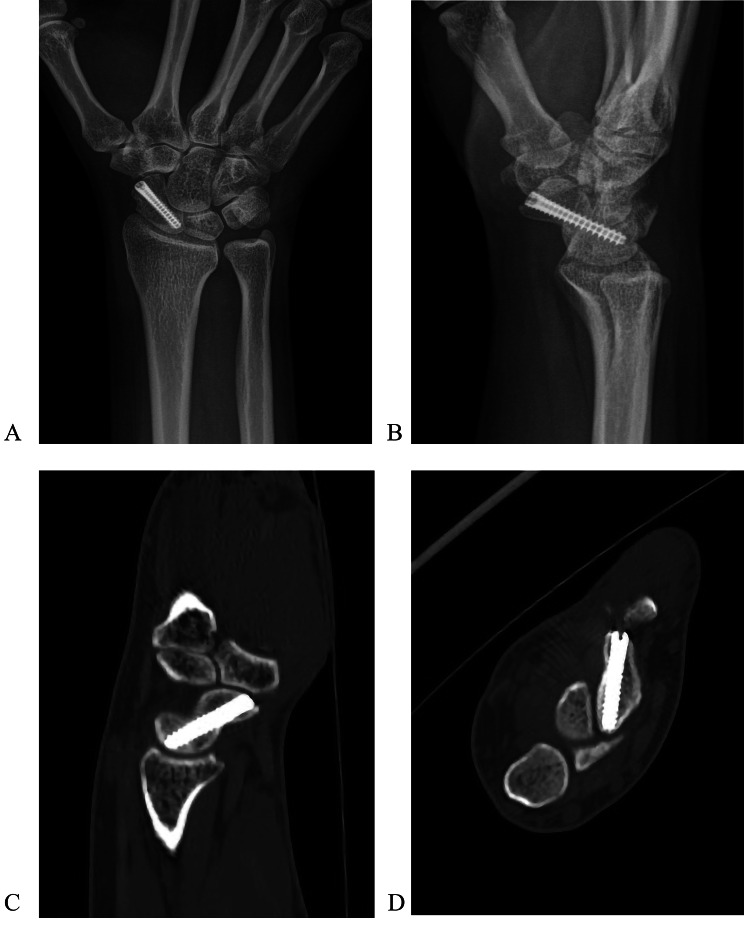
Anteroposterior (A), lateral (B) radiographs, sagittal (C), and horizontal (D) CT at 2 months postoperatively showed that the fracture was healed, and the screw was positioned in the most central region of the scaphoid
